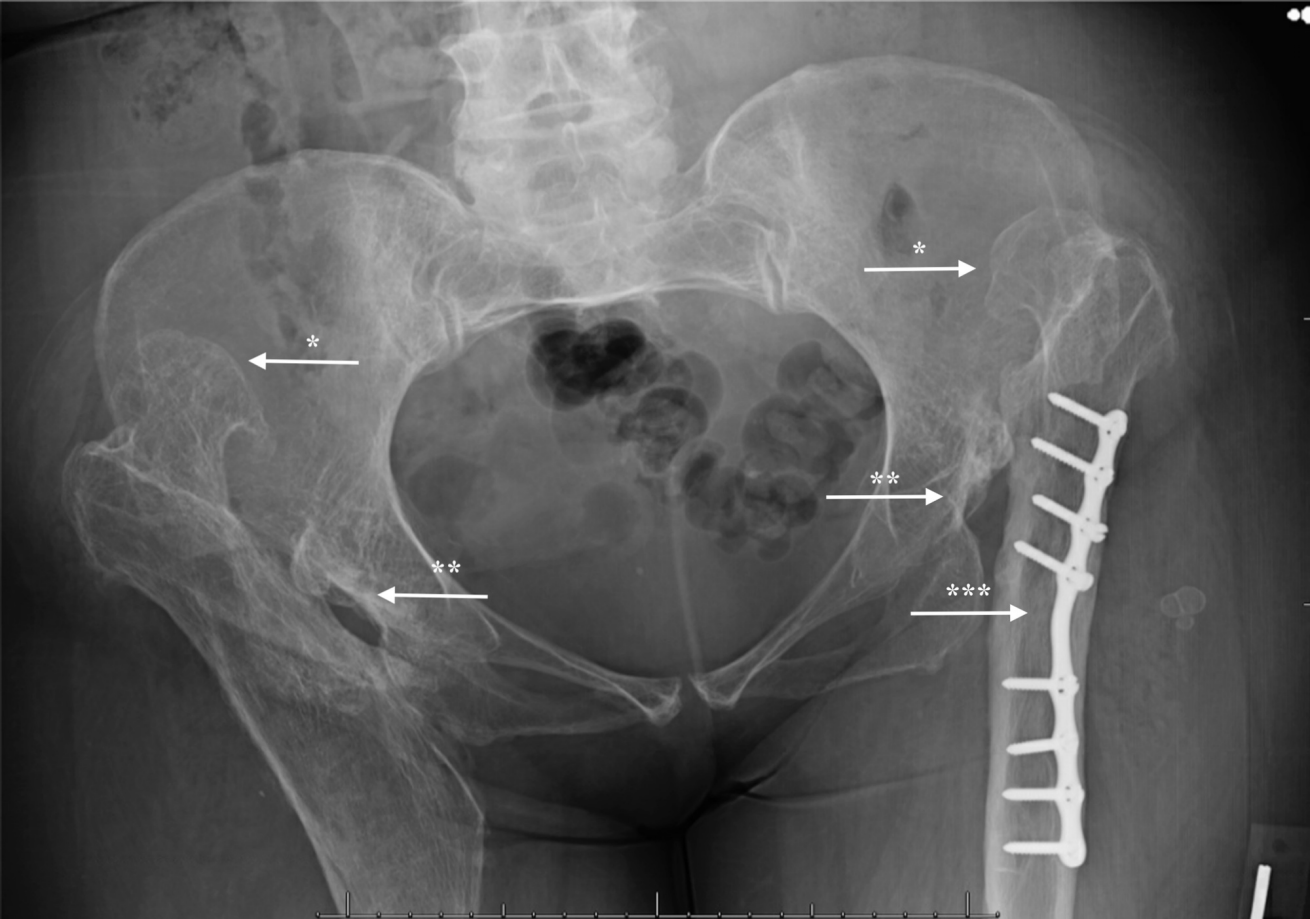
Anteroposterior view showing bilateral femoral dysplasia The femoral head and neck are dysplastic (*) with coxa valga deformity (increased caput collum diaphyseal angle/femoral neck anteversion angle), shallow acetabular socket () and femoral plate (*). X-rays: courtesy of Beaumont Royal Oak Hospital.
